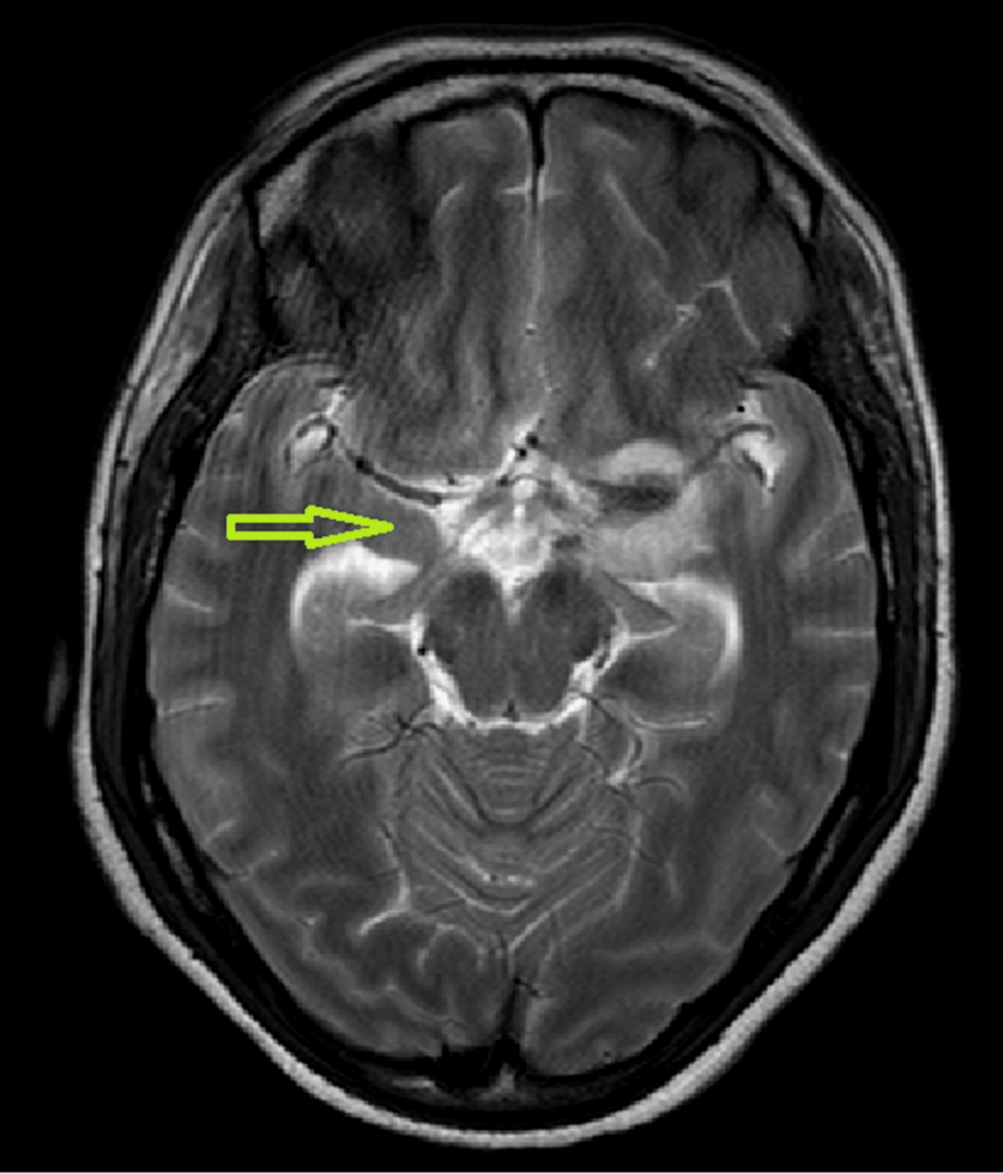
Axial MRI T2 image showing basal exudates and leptomeningeal enhancement
